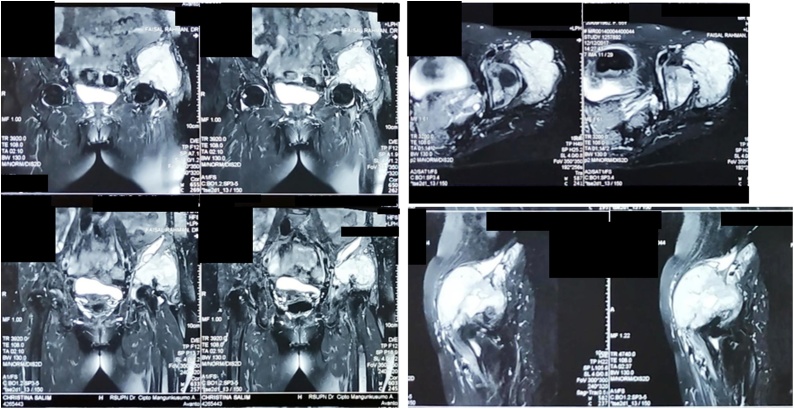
MRI Examination of the Pelvic.
